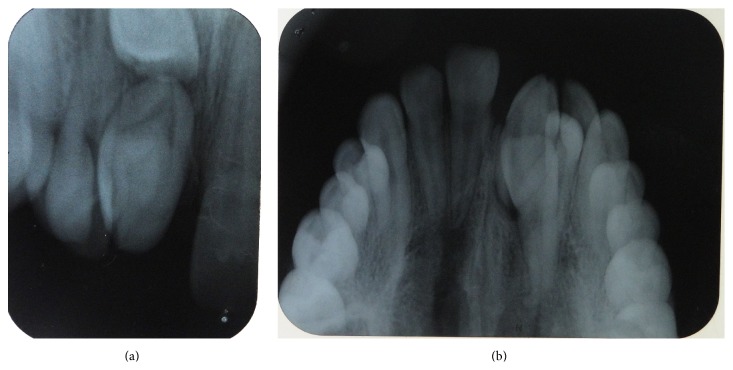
(a) Intraoral periapical radiograph and (b) anterior maxillary occlusal radiograph showing an erupted dilated odontoma with impacted central incisor.
